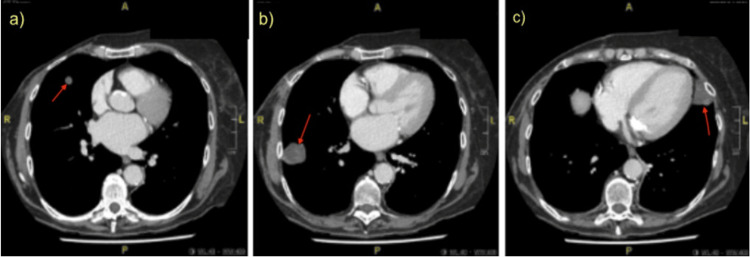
CT chest performed two months after mastectomy. Axial slices show two right lung nodules, measuring (a) 1.2 cm in the upper lobe and (b) 3.3 cm in the lower lobe. (c) A 3.3 cm lesion in the lingular segment of the left lung CT: computed tomography
